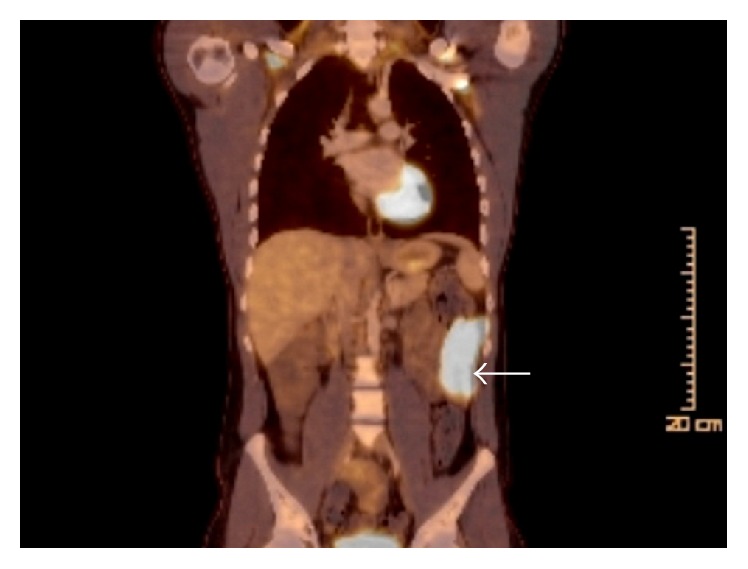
PET scan shows FDG avid small bowel segment with circumferential thickening (arrow) with no evidence of distant disease.
